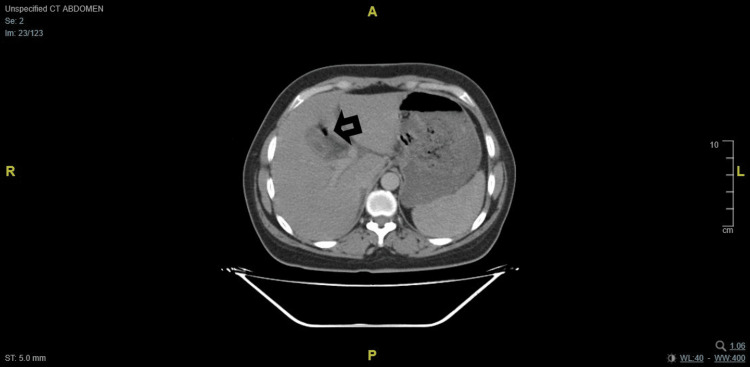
CT showing a distended gallbladder.
